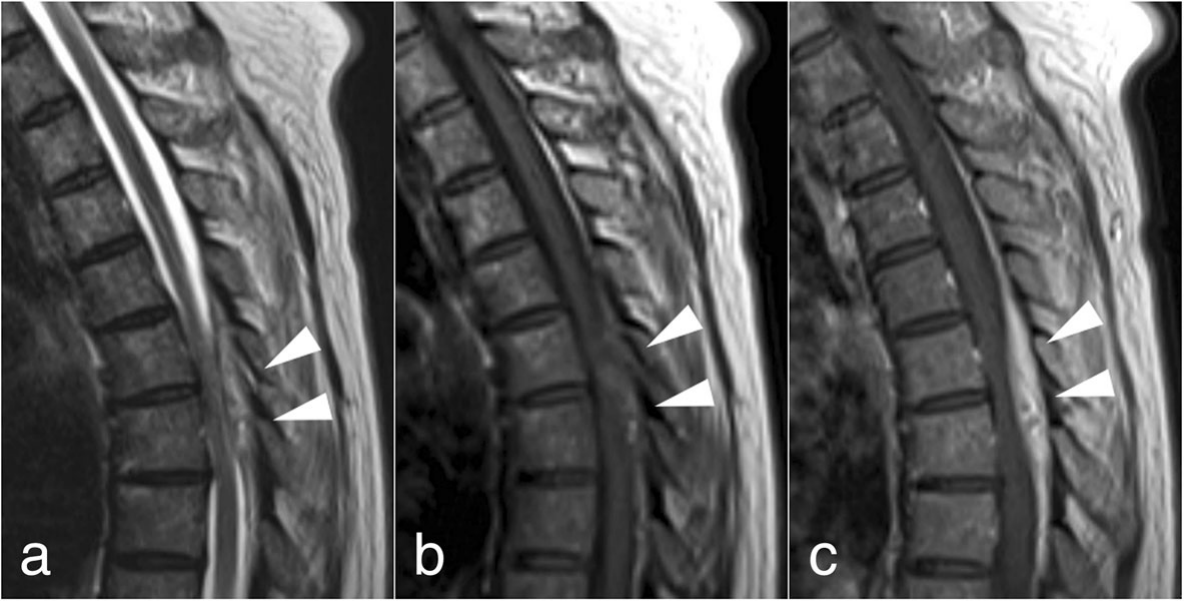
Sagittal MRIs of the thoracic spine on the eighth hospital day. a T2-weighted image. b T1-weighted image. c T1-weighted image with gadolinium enhancement. The posterior epidural abscess extends from the Th5 level to the Th6 level (arrow). Internally, there is a high T2/fluid signal, low T1 and gadolinium-enhancement. An associated mass effect caused spinal canal stenosis and slight compression of the spinal cord, without cord signal changes
